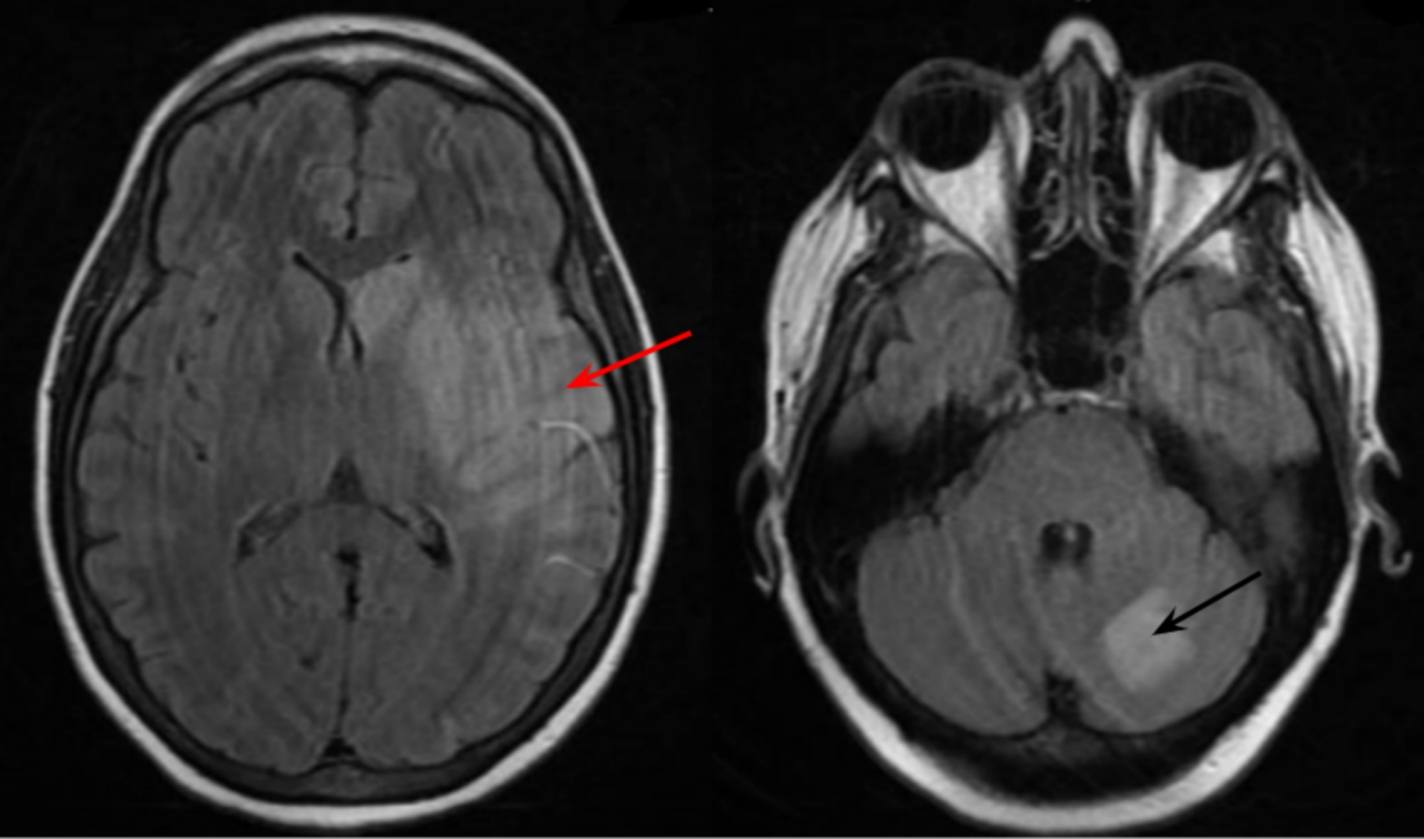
Magnetic resonance imaging of the brain demonstrating multiple large and small infarcts of the left parietal lobe and basal ganglia (red arrow) with an older infarct of the left cerebellum (black arrow) consistent with thromboembolic disease.
